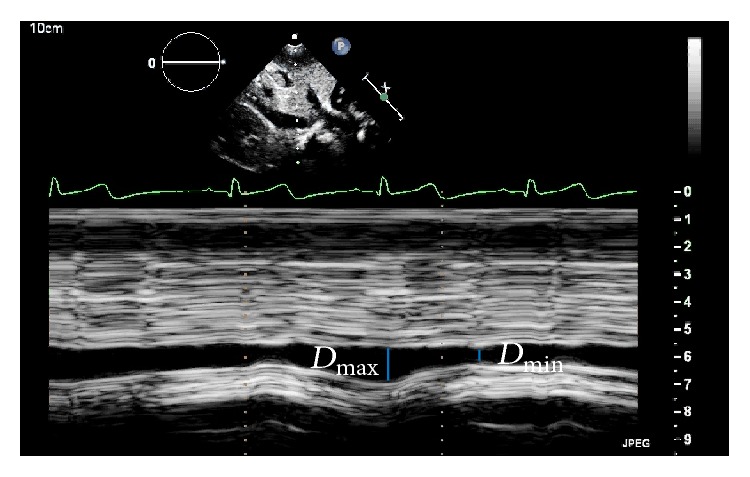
M mode echocardiography from subcostal view in a five-year-old patient in septic shock for urinary tract infection under mechanical ventilation, with sustained hypotension after volume expansion with 60 mL/kg of saline solution. Bedside echocardiography showed significant respiratory changes in IVC diameter, which, along with other clinical and monitoring data, suggested that fluid resuscitation should be maintained. dIVC = 90%, where dIVC = (*D*
